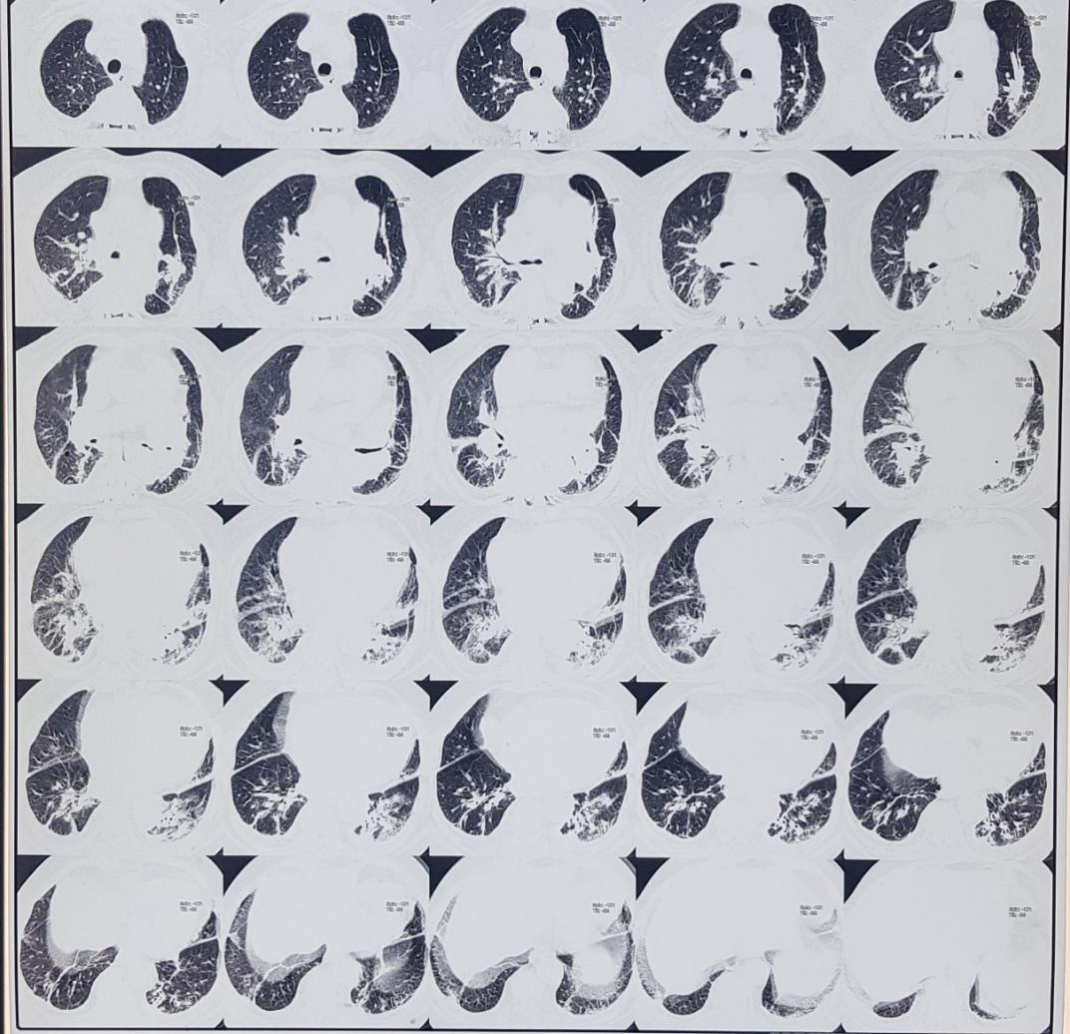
The multidetector-computed tomography scan of chest showing patchy consolidation with fibrotic bands.
